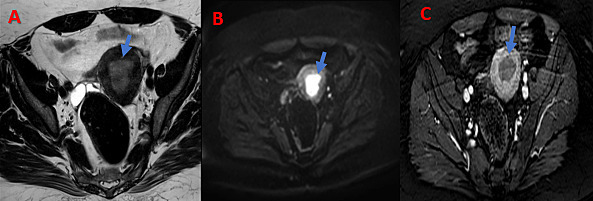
Pelvic MRI in the axial section of the T2 (A), diffusion (B) and T1 sequence after gadolinium (C) showing thickening of the tumor of the endometrium (blue arrow).
